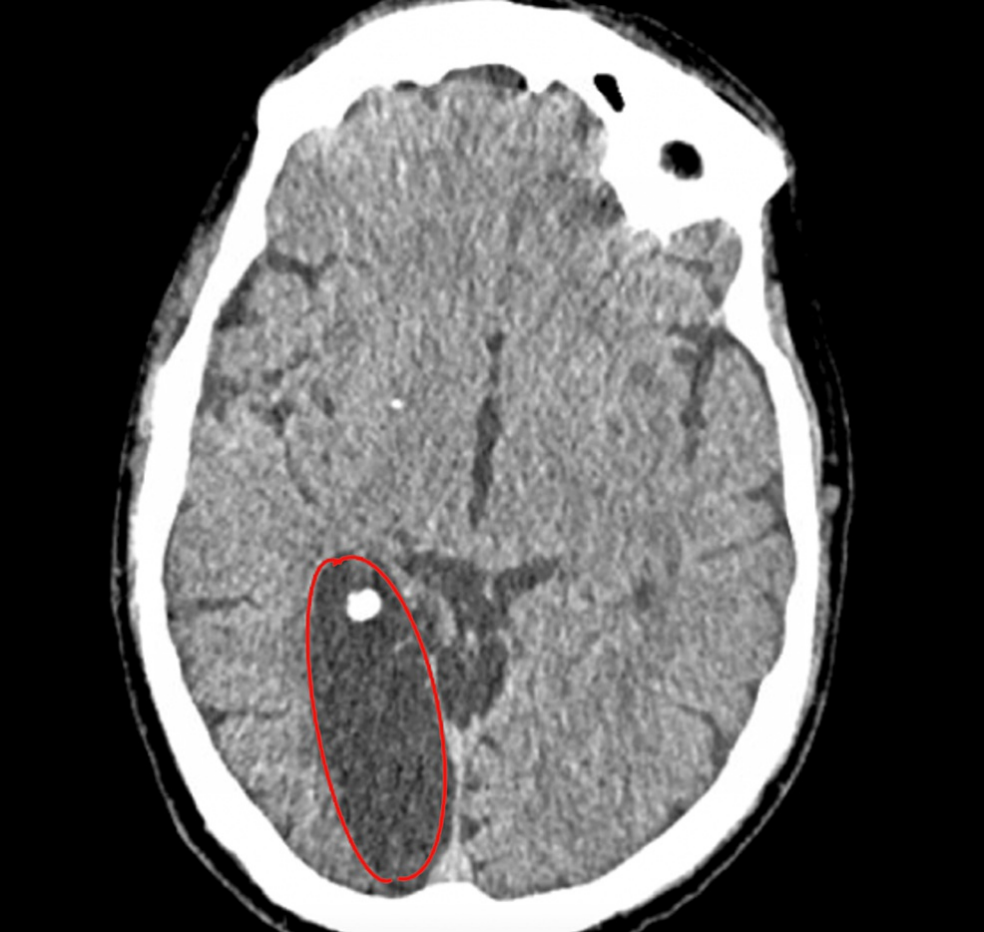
Non-contrast CT of the head showing the presence of encephalomalacia in the right posterior cerebral artery territory (red outline) due to a remote infarct
